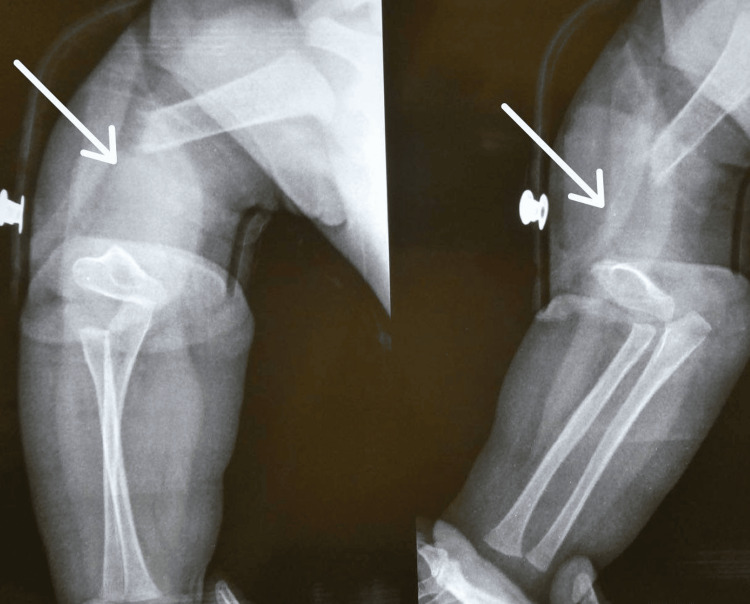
Preoperative radiograph demonstrating the segmental diaphyseal defect of the right humerus
